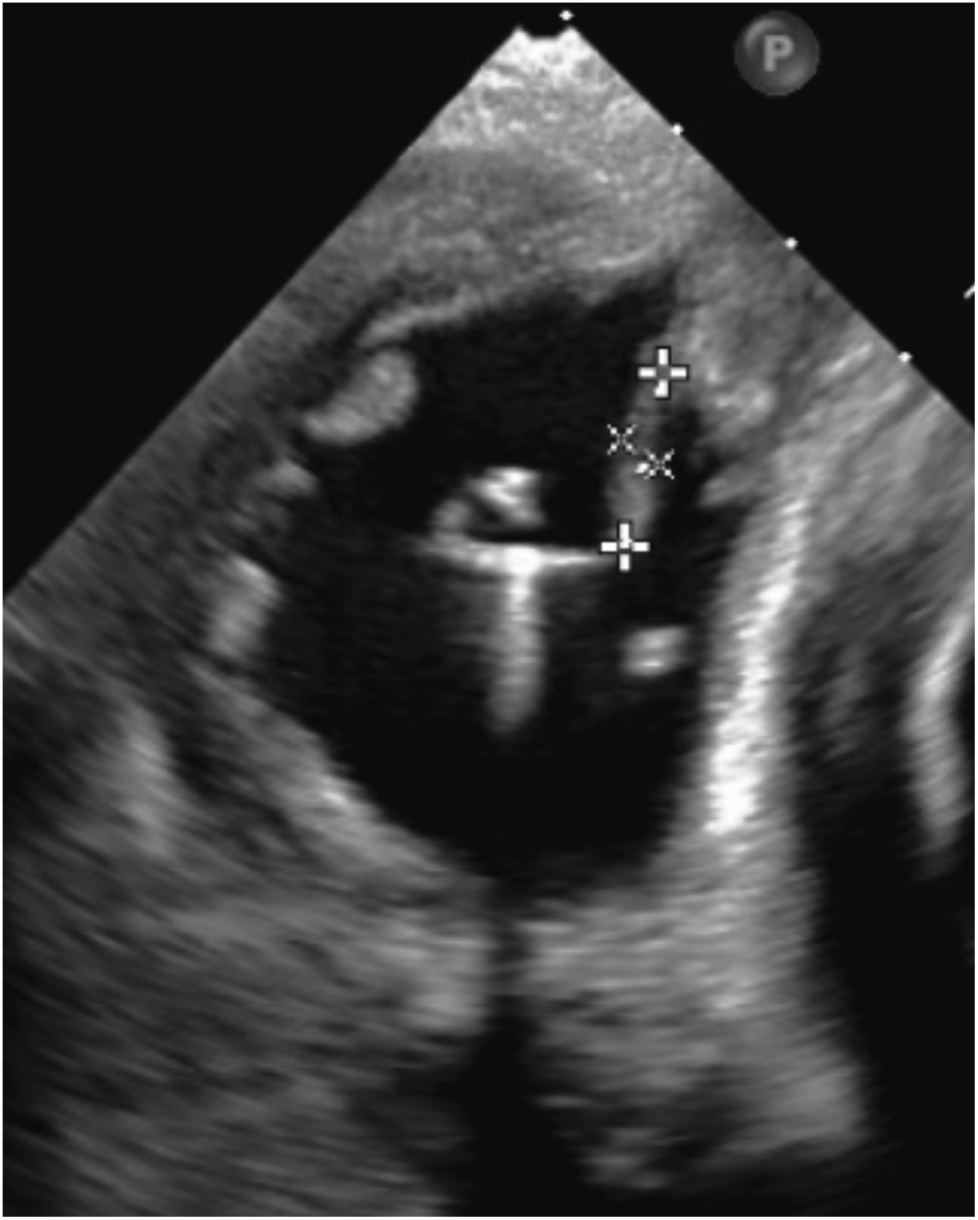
Transesophageal echocardiography showing vegetation.
